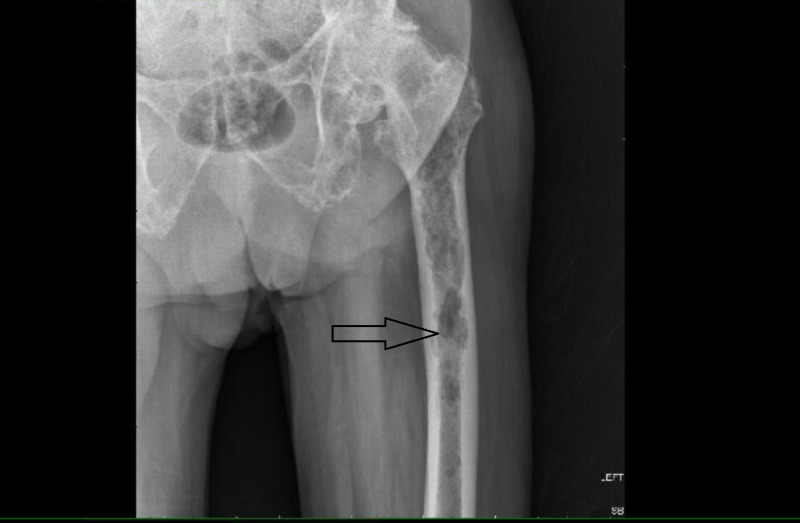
X-ray of the femur Showing multiple lytic lesions; arrow points towards one big lytic lesion.
